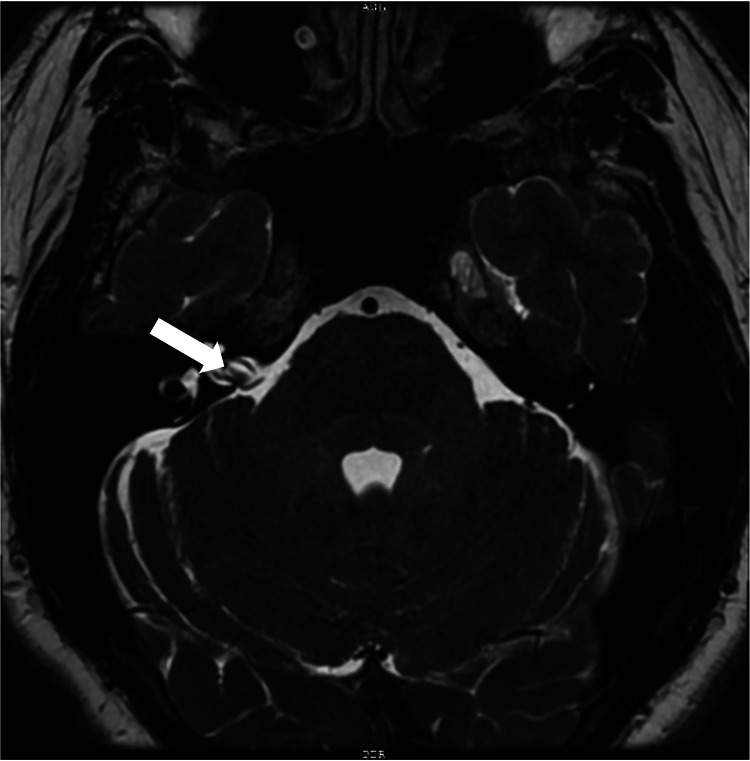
Multiplanar brain MRI with IV contrast indicates AICA loop against the vestibulocochlear nerve with no compression (white arrow). AICA, anterior inferior cerebral artery
